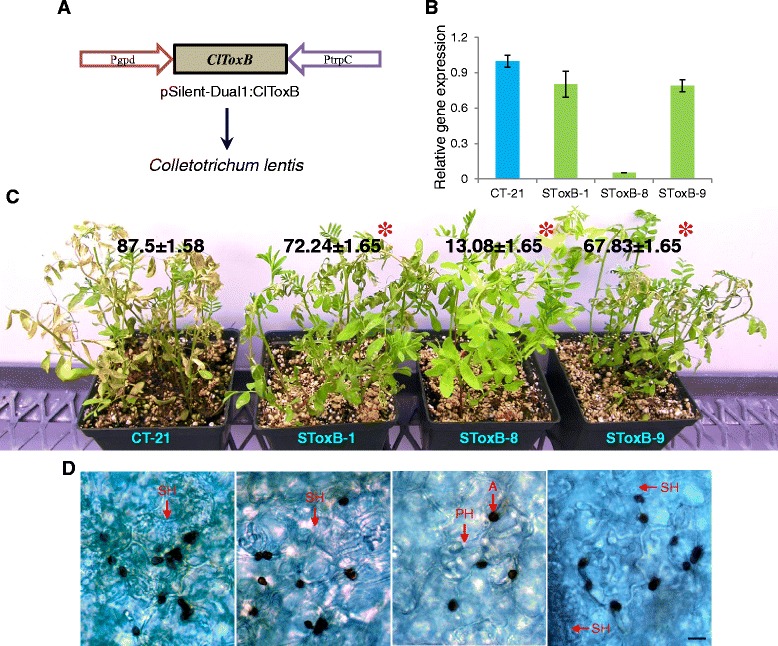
ClToxB* silencing. a RNA-silencing vector pSilent-Dual 1 (Nguyen et al. [55]). It contains two convergent promoters (Pgpd and PtrpC) of *Aspergillus nidulans. ClToxB* (JZ350031) was cloned in-between Pgpd and PtrpC promoters of pSilent-Dual 1, which was then used to transform *Colletotrichum lentis* isolate CT-21. b
